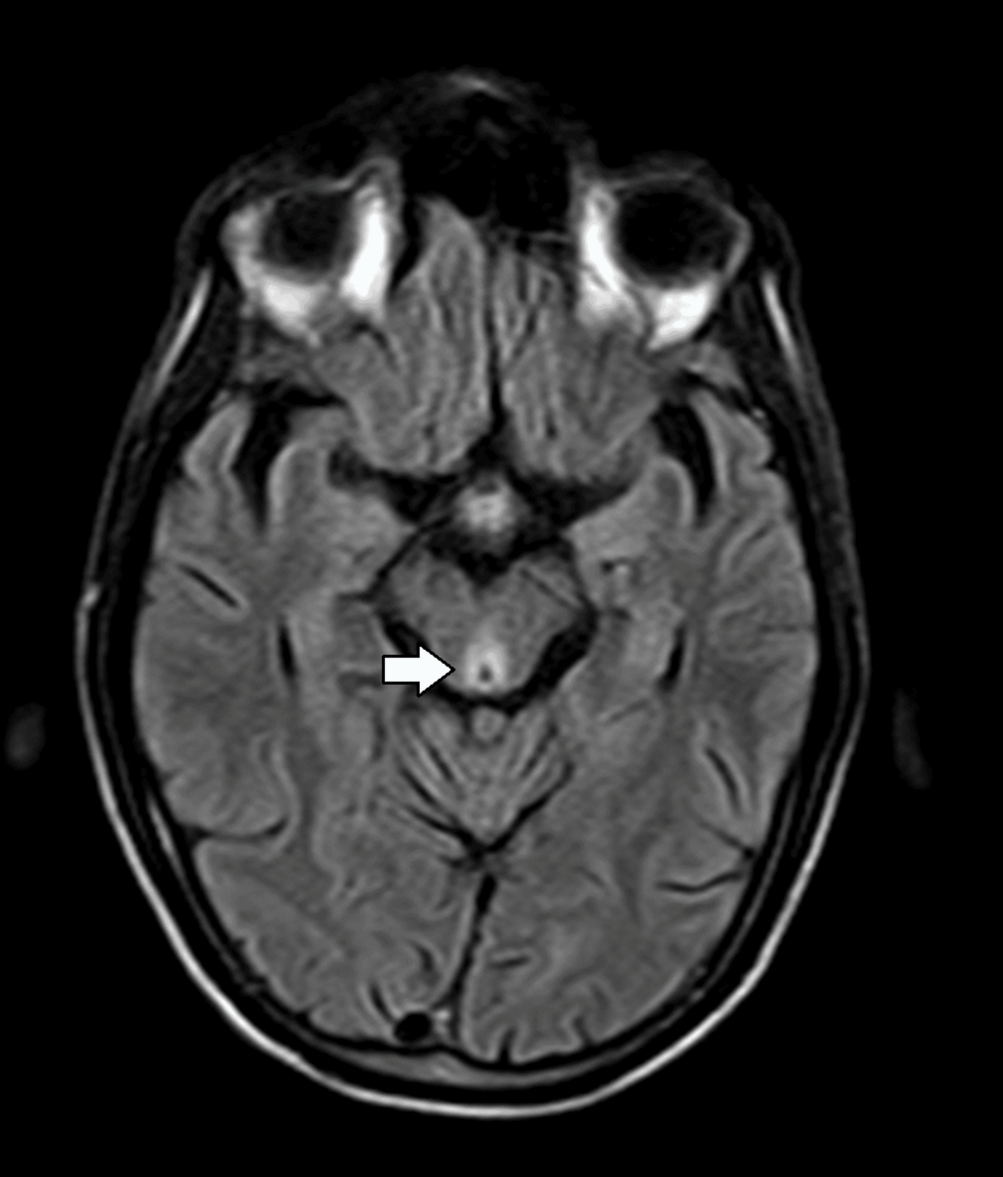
Axial FLAIR magnetic resonance image of the brain The axial FLAIR image shows a hyperintense signal in the white matter surrounding the third ventricle (arrow), consistent with involvement of periventricular regions commonly affected in WE. FLAIR: fluid-attenuated inversion recovery; WE: Wernicke encephalopathy
